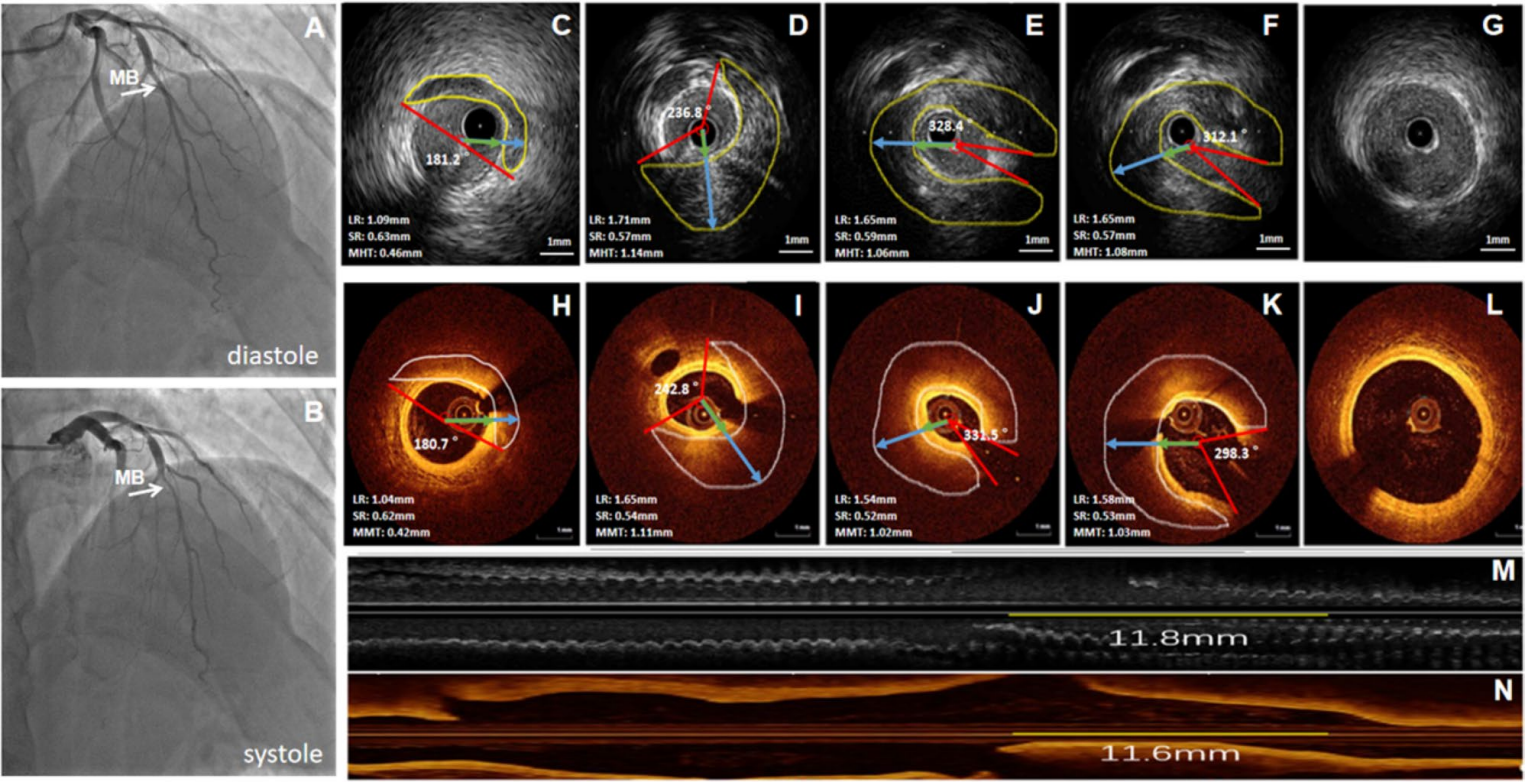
The representative case with MB was shown to measure indexes about the "half-moon" area and the muscle layer around the vessel's adventitia. The male patient with MB was 53 years old, and LAD was checked with CAG, IVUS, and OCT. (A,B) CAG results of MB. MB was located in the middle segment of LAD. The MLD in the MB segment was 2.43 mm during diastole and 0.97 mm during systole, and the compression rate of MB was 60%. (C–G,M) IVUS results of MB. (C) Showed the MHT was 0.46 mm, and the HA was 181.2°. (D) Showed the MHT was 1.14 mm, and the HA was 236.8°. (E) Showed the MHT was 1.06 mm, and the HA was 328.4°. (F) Showed the MHT was 1.08 mm, and the HA was 312.1°. (G) Showed there was no "half-moon" area in the proximal cross-section. (M) Showed the total length of the "half-moon" area was 11.8 mm. (H–L,N) OCT results of MB. (H) Showed the MMT was 0.42 mm, and the MA was 180.7°. (I) Showed the MMT was 1.11 mm, and the MA was 242.8°. (J) Showed the MMT was 1.02 mm, and the MA was 331.5°. (K) Showed the MMT was 1.03 mm, and the MA was 298.3°. (L) Showed there was no muscle layer in the proximal cross-section. (N) Showed the total length of the muscle layer was 11.6 mm. Scale bar: 1 mm. *MB* myocardial bridge, *LAD* left anterior descending artery, *CAG* coronary angiography, *IVUS* intravascular ultrasound, *OCT* optical coherence tomography, *MLD* minimum lumen diameter, *LR* long radius, *SR* short radius, *MHT* maximal "half-moon" layer’s thickness, *HA* "half-moon" layer’s arch, *MMT* maximal muscle layer’s thickness, *MA* muscle layer’s arch.
